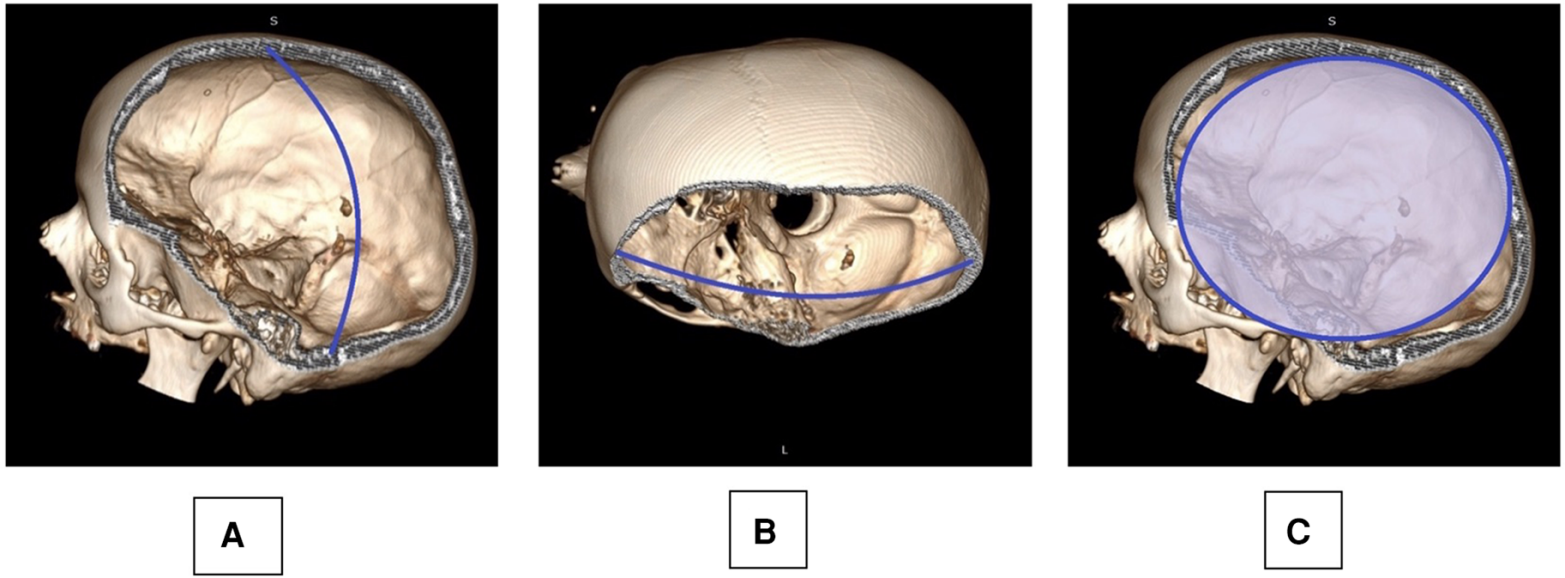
Skull reconstruction based on a postoperative CT scan is provided: the maximum cranio-caudal (A: CC) and anterior-posterior (B: AP) diameter were measured, taking into account also the anatomical curvature of the skull as observed on the contralateral side. A surface estimate of the decompression (C: SE) was deducted from the AP and CC measurements: SE=AP/2×CC/2×π.
